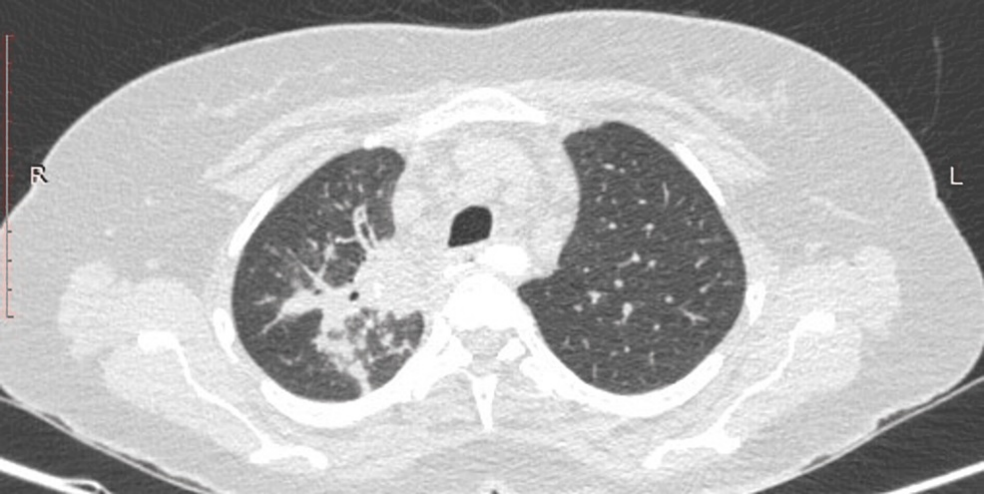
Computed tomography (CT) chest CT chest showing consolidation in right upper zone.
